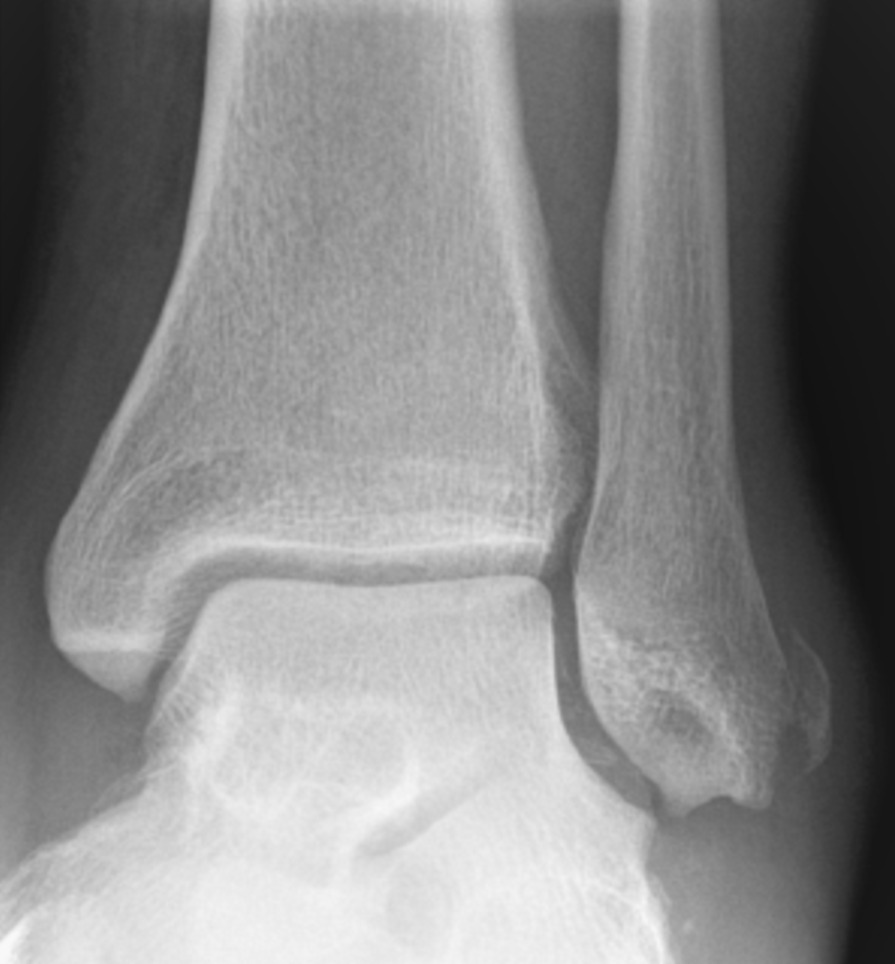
Mortise view x-ray showing a pathognomonic bony avulsion next to the lateral malleolus, the pathognomonic “fleck-sign”
